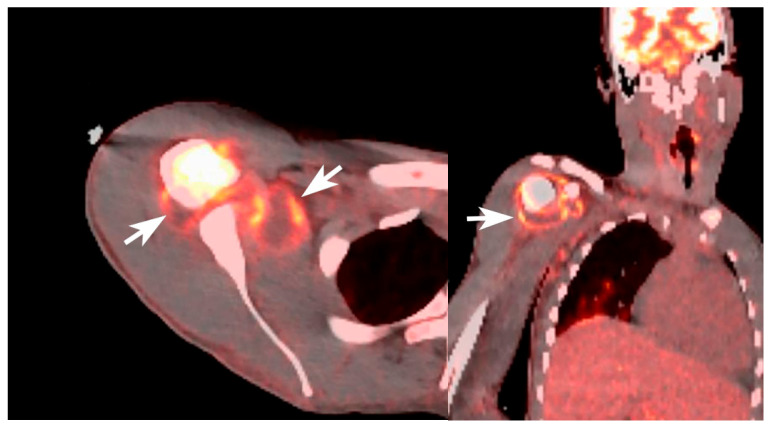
Axial and coronal PET-CT images demonstrating abnormal hypermetabolism involving the proximal right humerus and peripheral hypermetabolism surrounding the joint effusion. Arrows point to the inflamed (hypermetabolic) synovium surrounding the joint effusion in the setting of septic arthritis.
